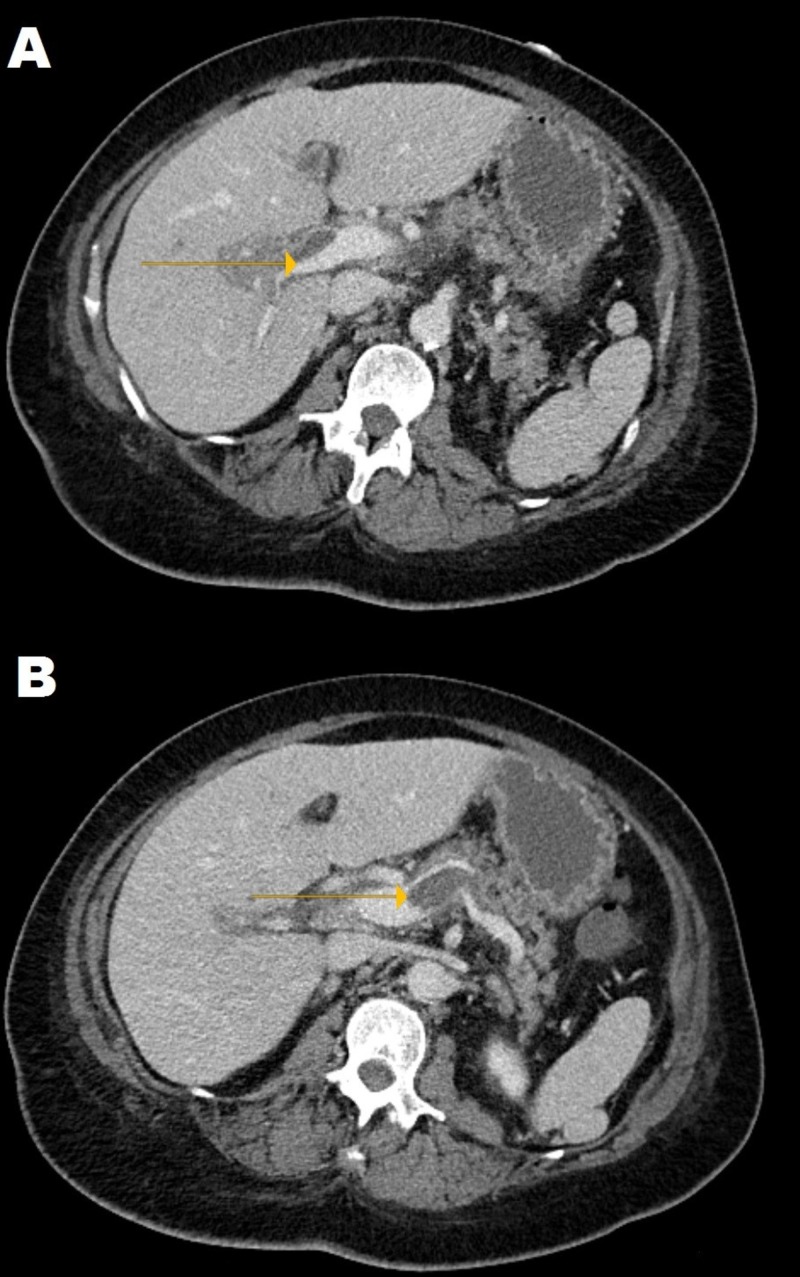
An abdominal computed tomography scan with contrast showing an acute portal vein thrombosis (A) extending into the splenic vein (B) and segmental branches of the right and left hepatic lobes.
